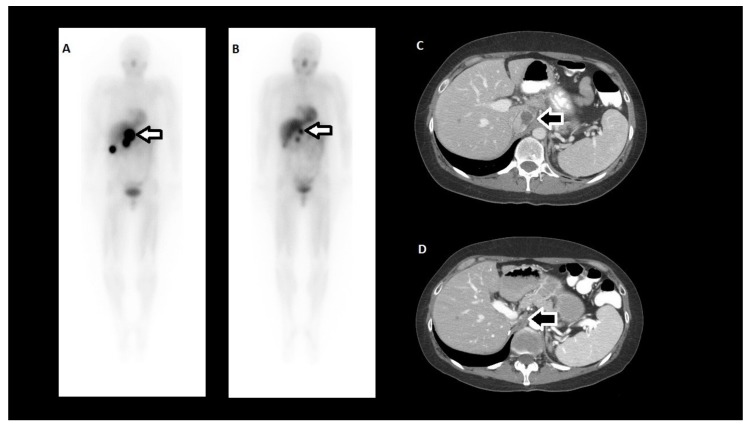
Clinical example of a tumor radiographic response to HSA-I-131-MIBG in a patient treated at The University of Texas MD Anderson Cancer Center. (A) HSA-131-MIBG scan 3 days after the first treatment; (B) HSA-131-MIBG scan 3 days after the second treatment; (C) Computed tomography prior to the first HSA-131-MIBG treatment; (D) Computed tomography 11 months after the second HSA-131-MIBG treatment (44% reduction in longest tumor dimension). Arrows indicate the tumor.
